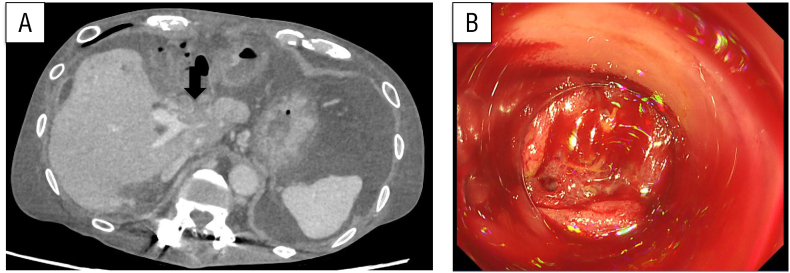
(A) Computed tomography showed portal vein stenosis caused by tumor recurrence (arrow). (B) Enteroscopy revealed bleeding from varices at the choledochojejunostomy site.
